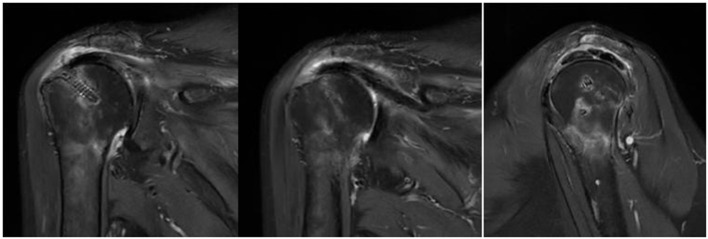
Sugaya's classification types I and II in postoperative MRI.
